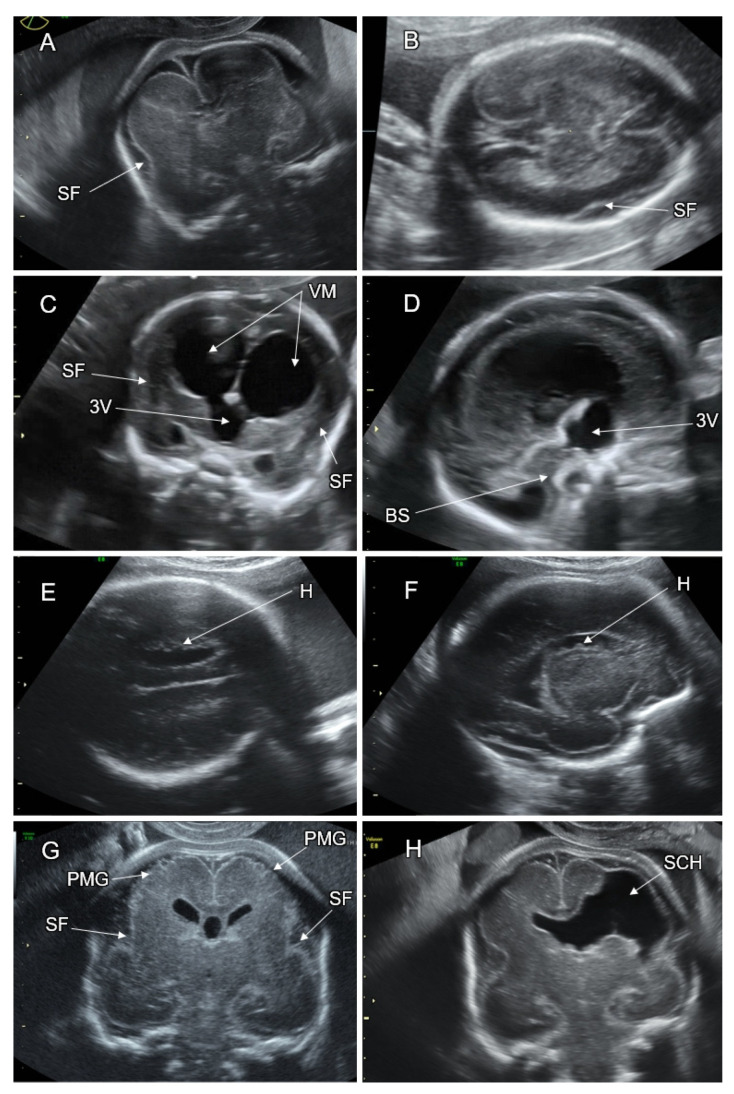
Fetal sonographic features of malformations of cortical development. Legend: (A,B) Transthalamic coronal and axal planes of a fetus with lissencephaly at 24 gestational weeks. Note the shallow Sylvian fissures (SF). (C,D) Transventricular axial and sagittal planes of a fetus with Walker–Warburg syndrome at 26 gestational weeks. Note the flat Sylvian fissures (SF), severe dilatation of the lateral (VM) and third (3V) ventricles, and the typical Z-shaped hypoplastic brainstem (BS). (E,F) Transventricular axial and sagittal ventricular planes of a female fetus with periventricular nodular heterotopia at 32 gestational weeks. Note the chain of the small subependymal protrusions bulging into the ventricle (PNH). (G) Transthalamic coronal plane of a fetus with bilateral frontal polymicrogyria at 25 gestational weeks. Note an irregular, serrated cortical contour (PMG). (H) Transthalamic coronal planes of a fetus with schizencephaly at 22 gestational weeks. Note the extensive lateral parenchymal cleft (SCH) extending from the cerebral cortex to the ventricle associated with the absence of the cavim septi pellucidi.
